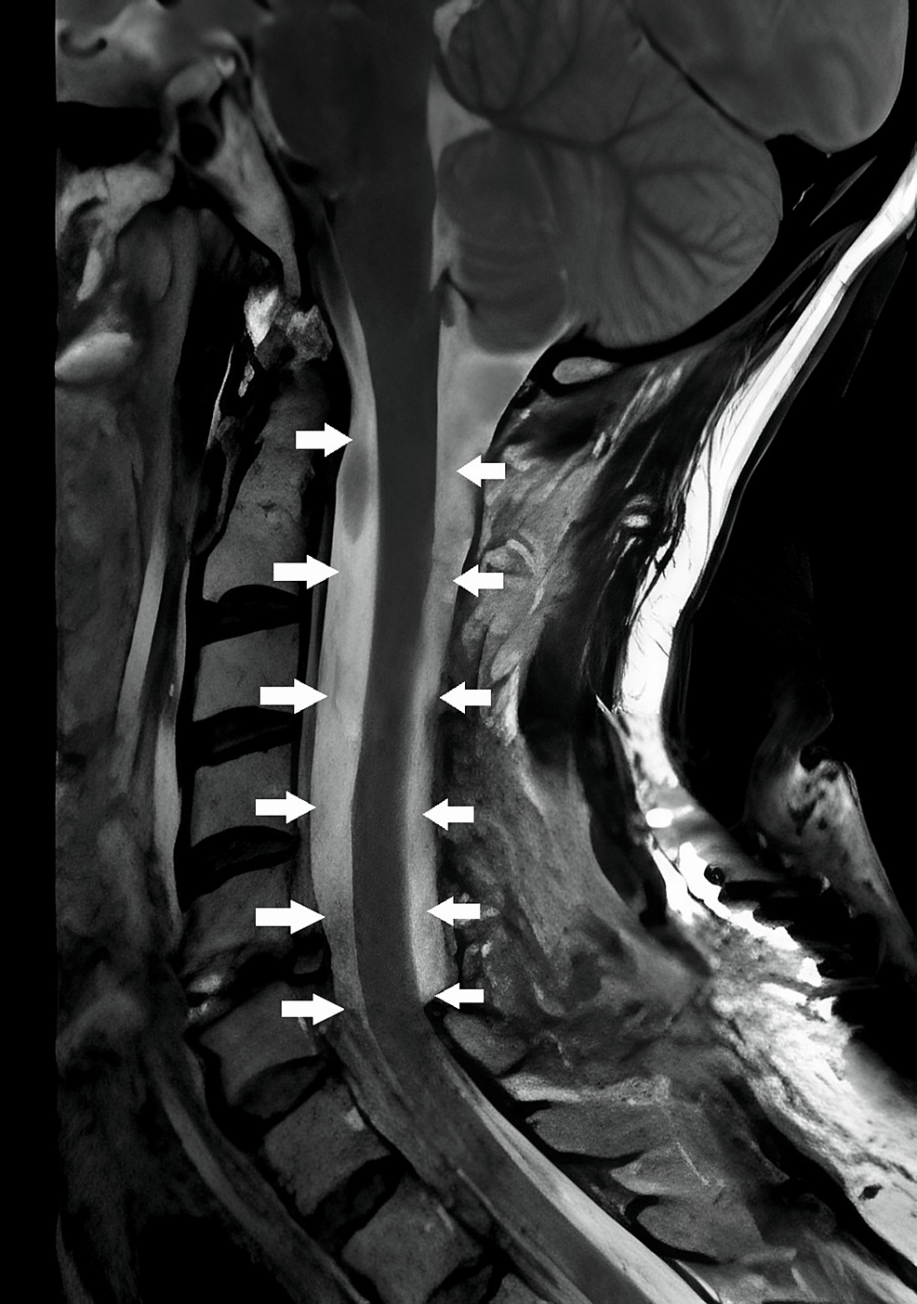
Sagittal T2-weighted MRI one day after the surgery showing no abnormality in the medulla and the achieved decompression (white arrows).
